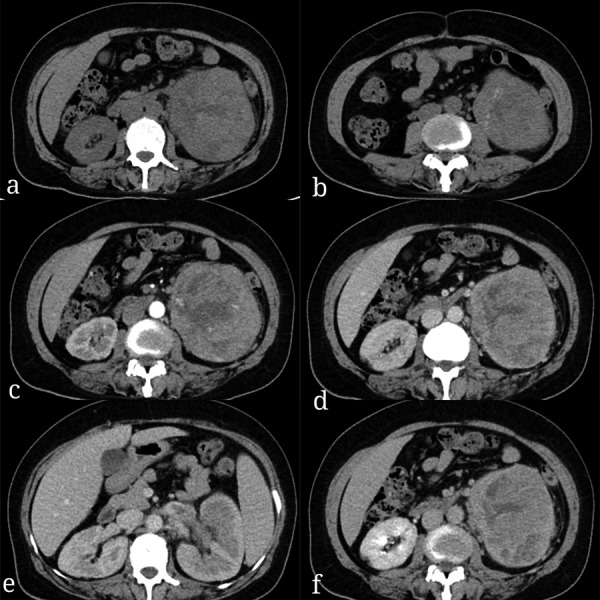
a, b: Precontrast computed tomography images showed a heterogeneous density mass with spot calcification. c: The mass showed moderate heterogeneous enhancement on corticomedullary phase with necrosis and many tumor vessels. d: The tumor showed continued enhancement on the nephrographic phase and (f) slight washout on the delayed phase. e: The filling defect in the left renal vein and enlarged retroperitoneal lymph nodes are shown.
